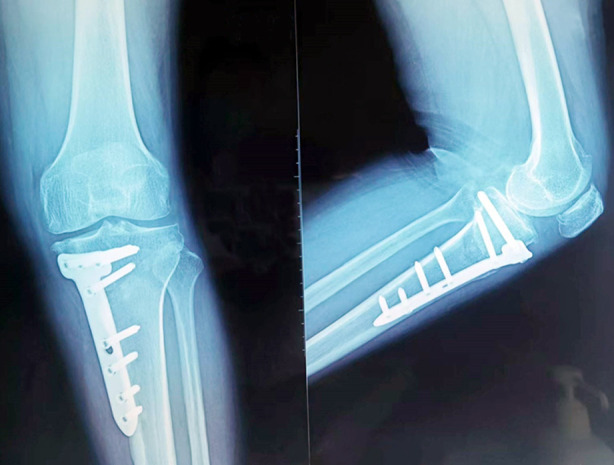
Radiographs of patients with medial knee osteoarthritis after HTO combined with arthroscopy.
